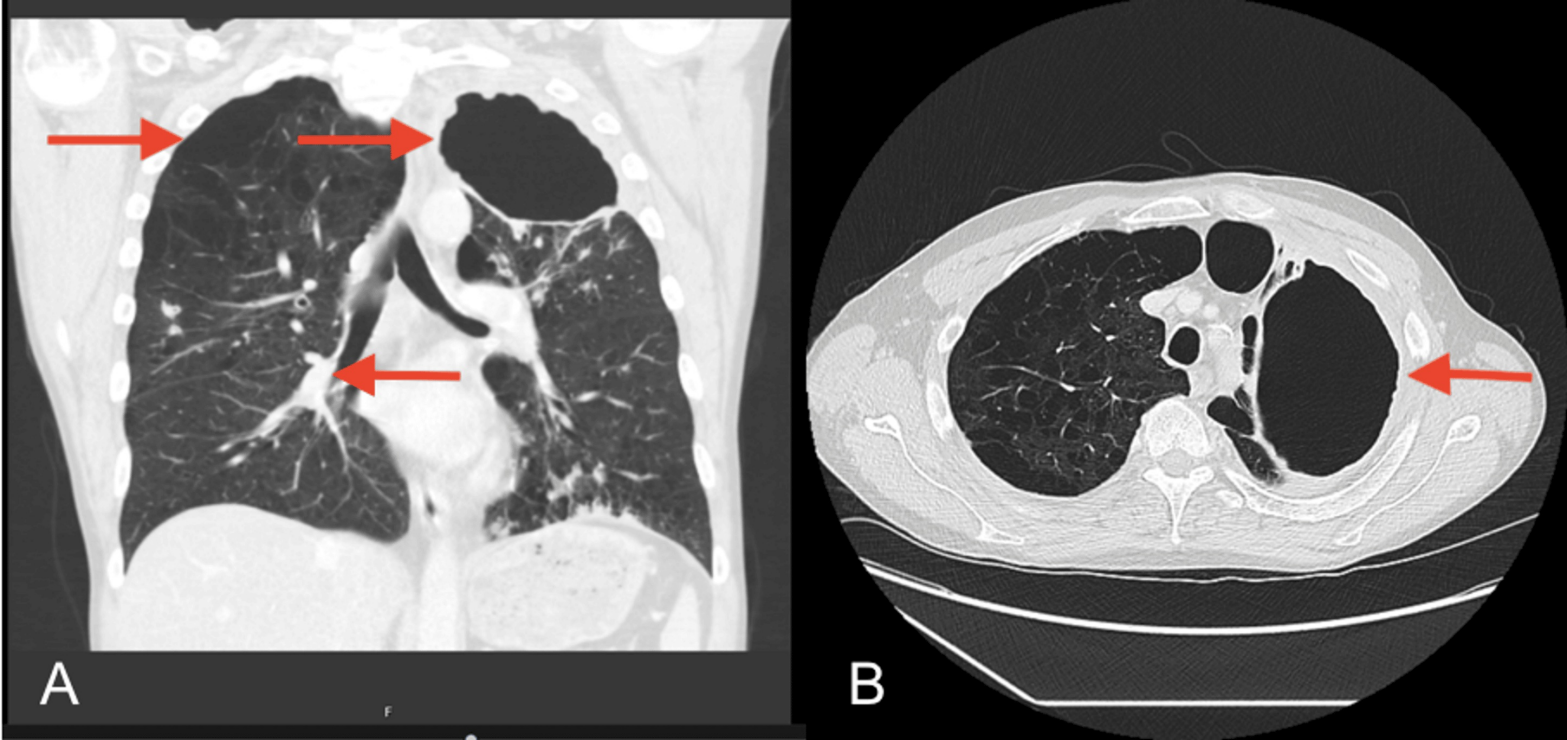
Computed tomography (CT) scan Image A highlights a large chronic cavitating lesion in the left upper lobe, marked by an arrow, with progressive nodular wall thickening. Additional arrows point to bilateral inflammatory nodules, tree-in-bud changes in both upper lobes, and bullous emphysema. Image B presents a cross-sectional view of the same pathology, with an arrow indicating the large apical cavitating lesion.
